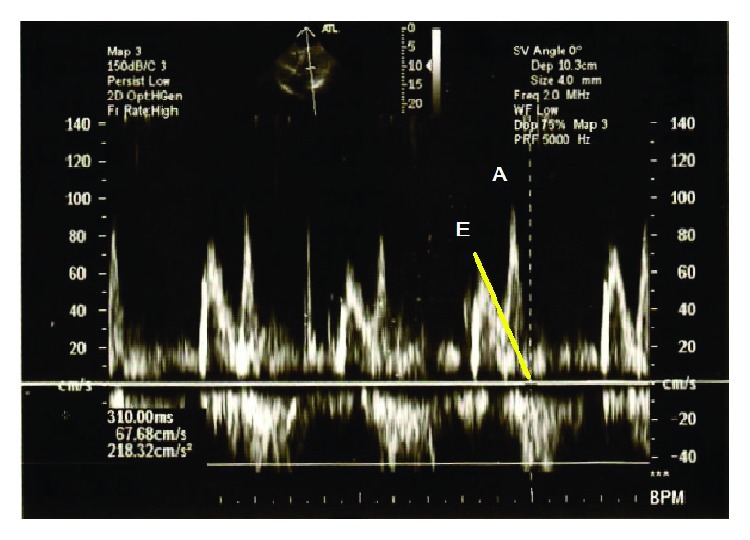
Diagnosing diastolic dysfunction with echocardiography. Transmitral flow velocities measured with pulsatile wave Doppler technique. The ratio (*E*/*A*) of the early diastolic peak velocity (*E*) and the late diastolic velocity (*A*) is lower than 1. Deceleration time (DT) is the interval from the peak of the wave *E* to its end (marked with a yellow line). In this case, its prolongation was measured (310 sec). The above alterations prove the left ventricular diastolic dysfunction (relaxation disorder).
